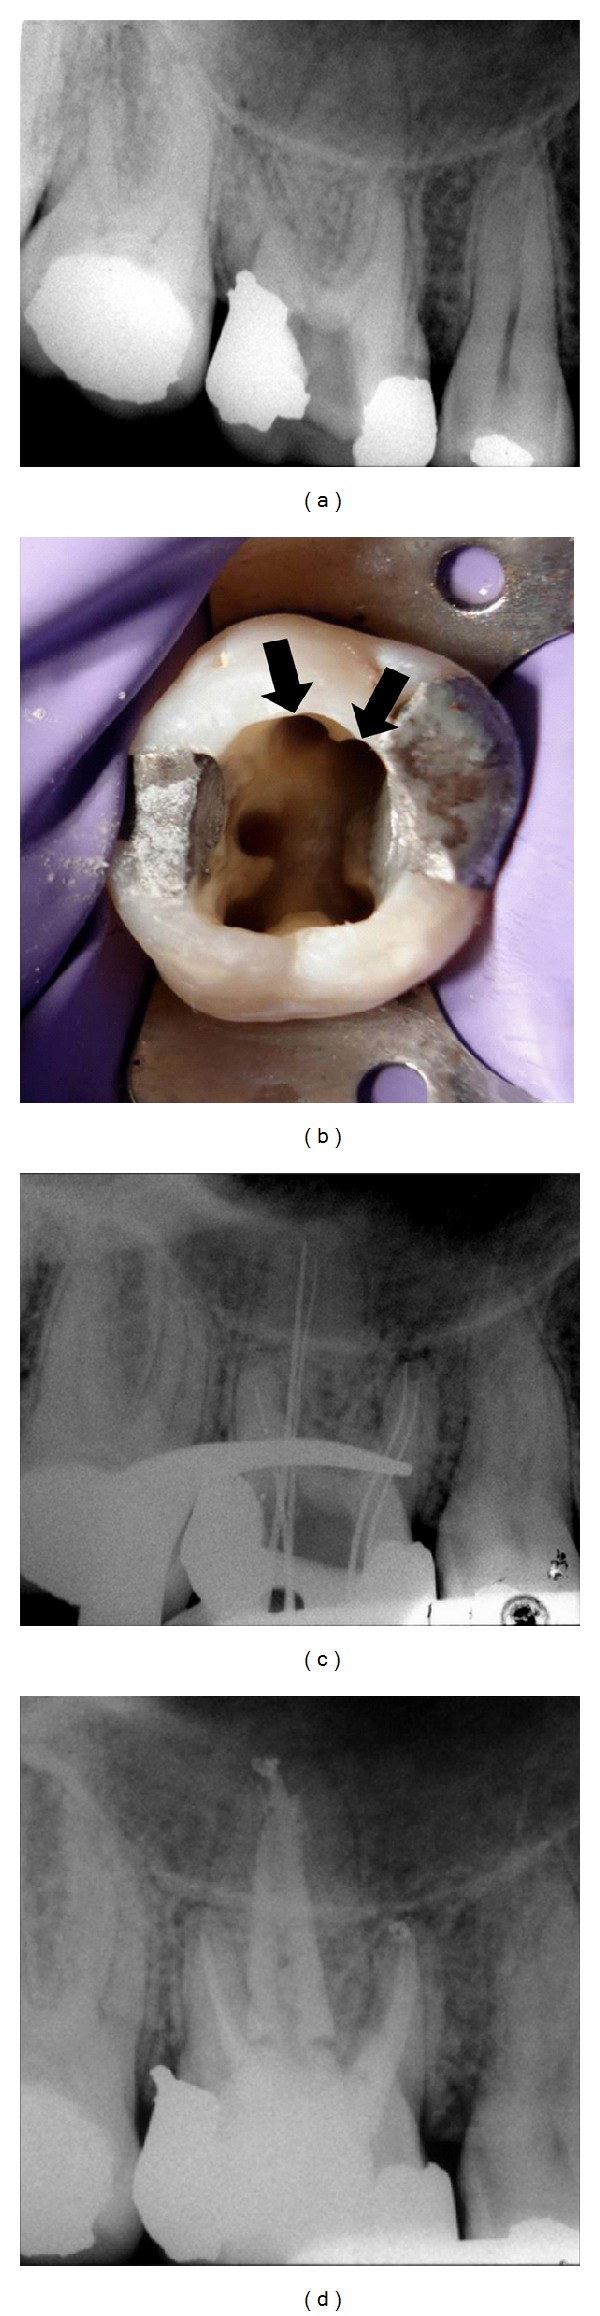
(a) Initial radiographic aspect of tooth number 3. (b) Endodontic access showing the presence of five root canal entrances. (c) Radiograph for working length determination. (d) Radiographic aspect after root canal fillings.
